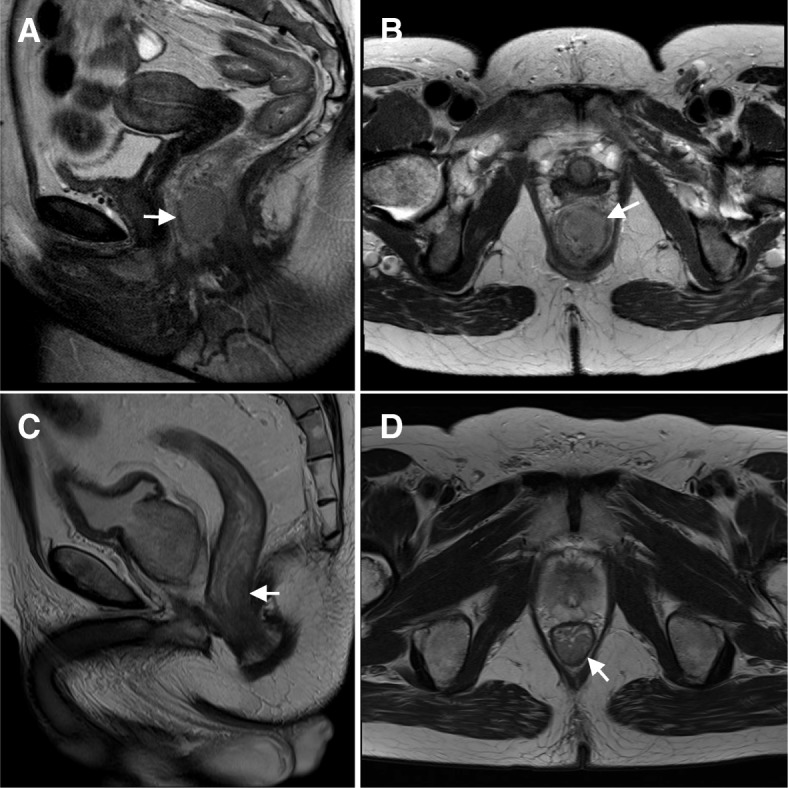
T2-weighted MRI of the pelvis of the female case shows a 3-cm lesion in the anal canal extending into the anorectal junction (a. sagittal, b. axial). MRI of the male case also demonstrates a T2 lesion measuring about 3 cm beginning at 2 cm from the anal verge (c. sagittal, d. axial)
